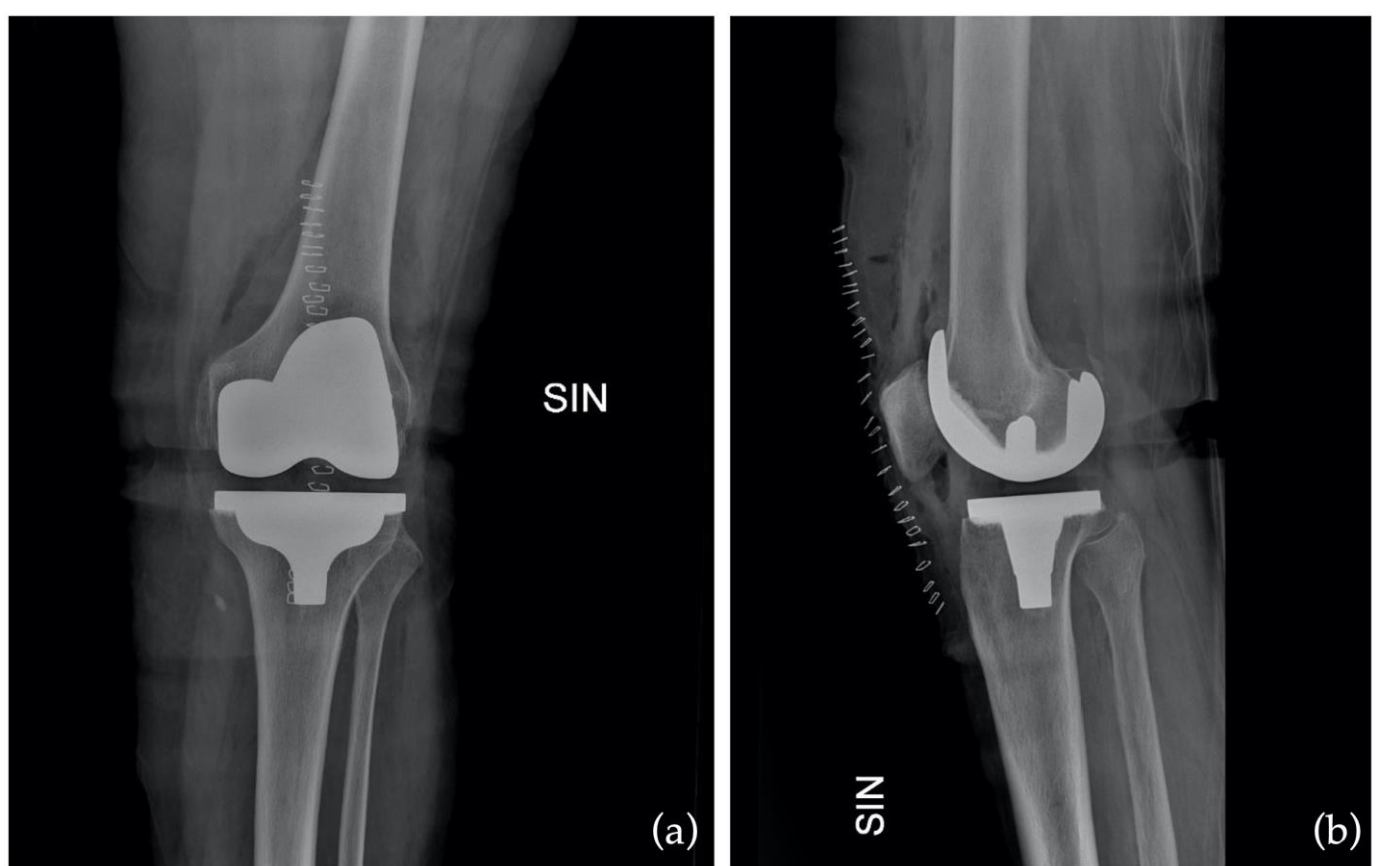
Postoperative X-ray in anteroposterior (AP) as (a) and in latero-lateral (LL) as (b).
